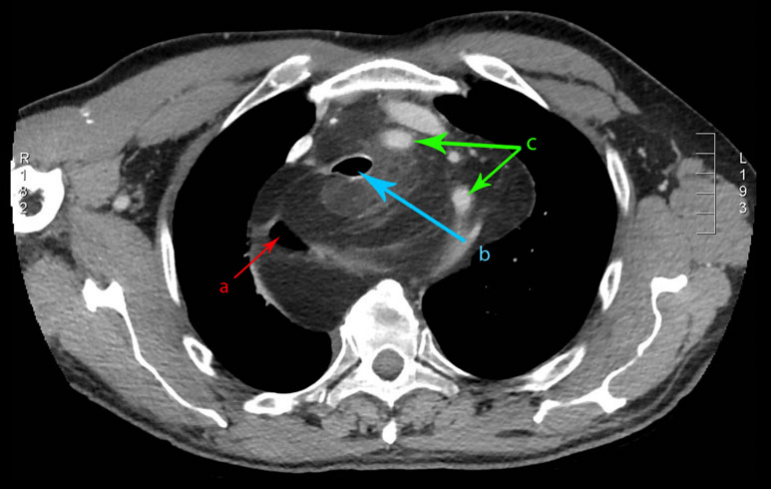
Axial CT image of the mediastinal liposarcoma. (a) indicates the position of the esophagus, (b) indicates the trachea, and (c) demonstrates the arch vessels.
